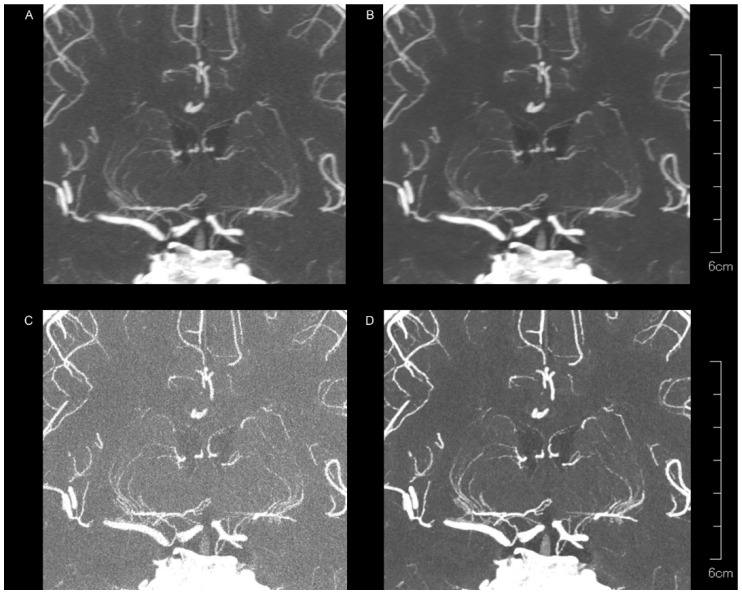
Representative UHR PCD-CTA images with coronal partial maximum intensity for a 68-year-old female, showing kernel Hv40 without QIR (A) and with QIR (B), as well as kernel Hv72 without QIR (C) and with QIR (D) in the LSA region. The LSAs are generally well visualized across all reconstruction methods, but vessel sharpness and image noise vary among methods.
